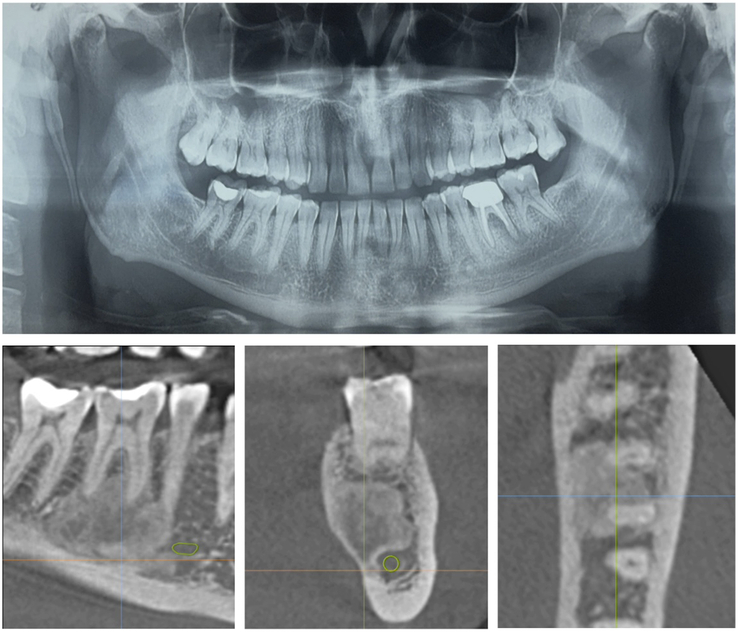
Radiographic images: orthopantomography, and coronal, axial, and panorex CBCT sections of the osteosclerotic lesion.
